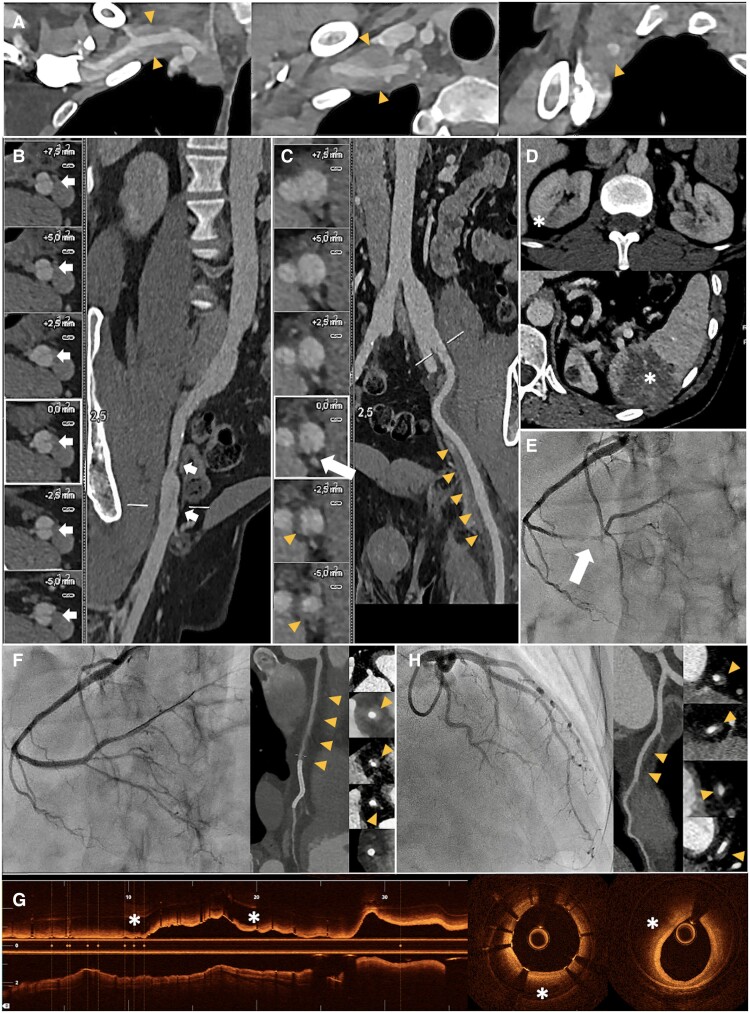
A 41-year-old male presented with abdominal pain following a prolonged flight. He was diagnosed with Ehlers–Danlos syndrome (EDS) by the COL3A1 gene mutation 8 years earlier, after a spontaneous dissection of the right common iliac artery during the course of a half-marathon. In the current admission, computed tomography (CT) angiography revealed a mural haematoma in the right subclavian artery *(Panel A)* and a known chronic dissection flap in the right external iliac artery *(Panel B)*. A flap was identified in the left external iliac artery, associated with concentric mural thickening suggestive of a haematoma *(Panel C, arrow and triangles, respectively)*. Bilateral kidney ischaemic lesions with repletion defects in the posterior branches of both renal arteries and a splenic ischaemic lesion *(Panel D)* were also noted. Conservative treatment was indicated.
